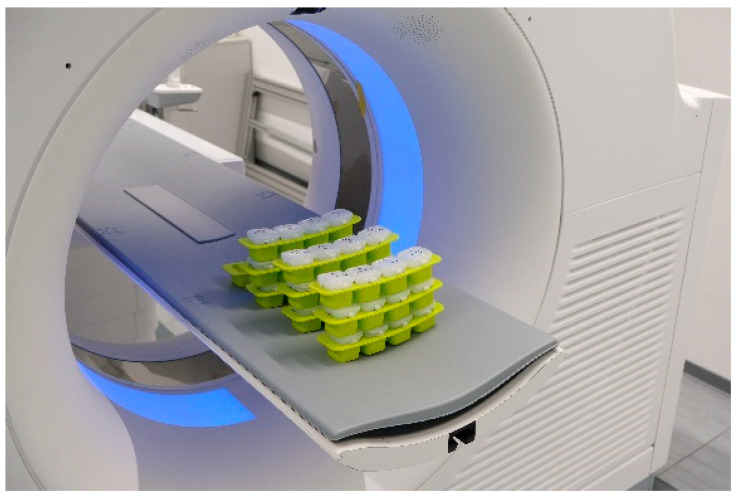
Sample container setup in the CT gantry.
